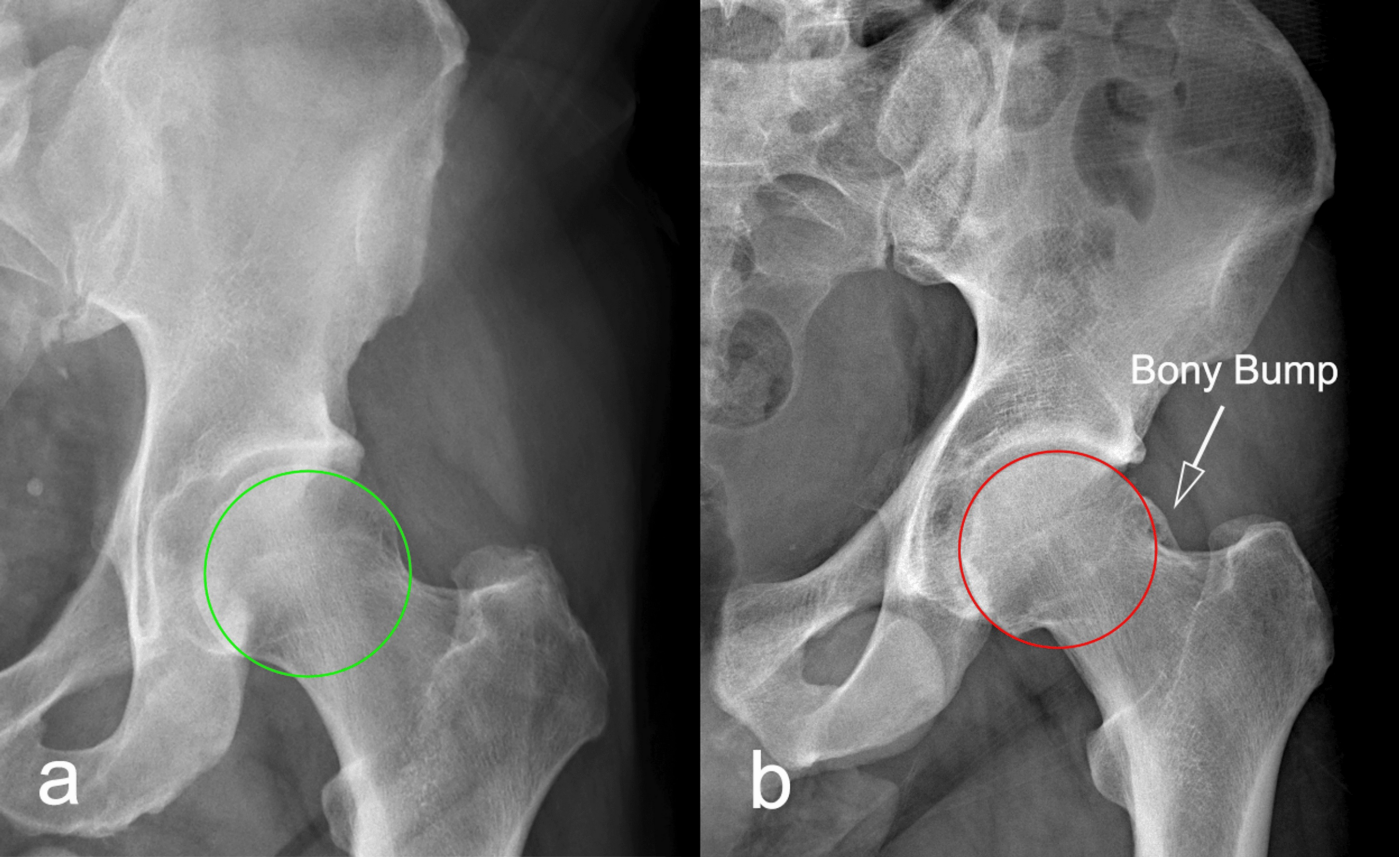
Coronal plane radiographs of hip a) Normal hip joint; b) hip joint showing a clear superior head-neck deformity, defined as a bony bump or pistol grip deformity. Image credit: Author, Saverio Colonna
